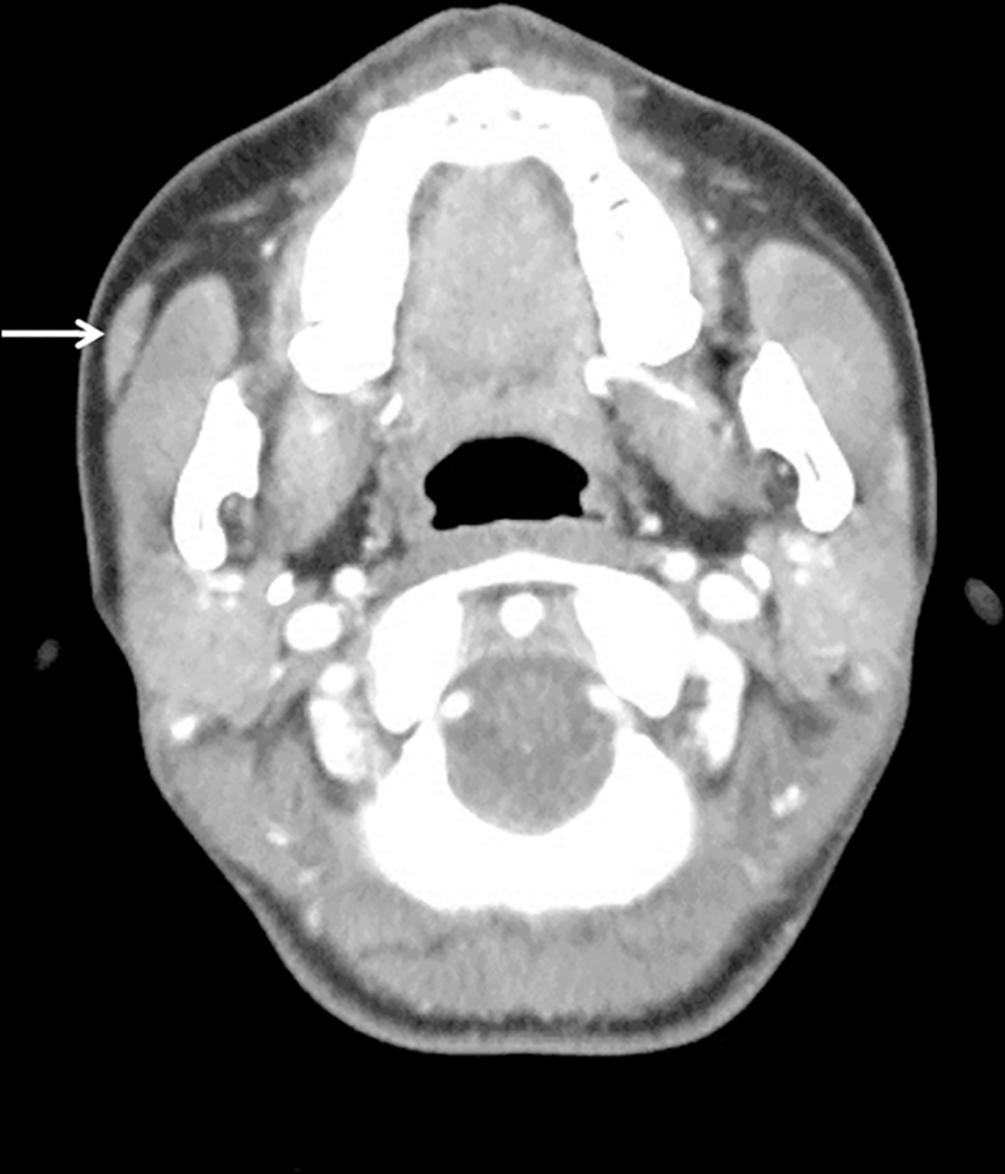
A representative example of an accessory parotid gland. A soft-tissue mass that has the same density as the main parotid gland is shown, is located at the anterior part of the main parotid gland, and is completely separate from the main parotid gland on every slice of the CT scan (arrow).
